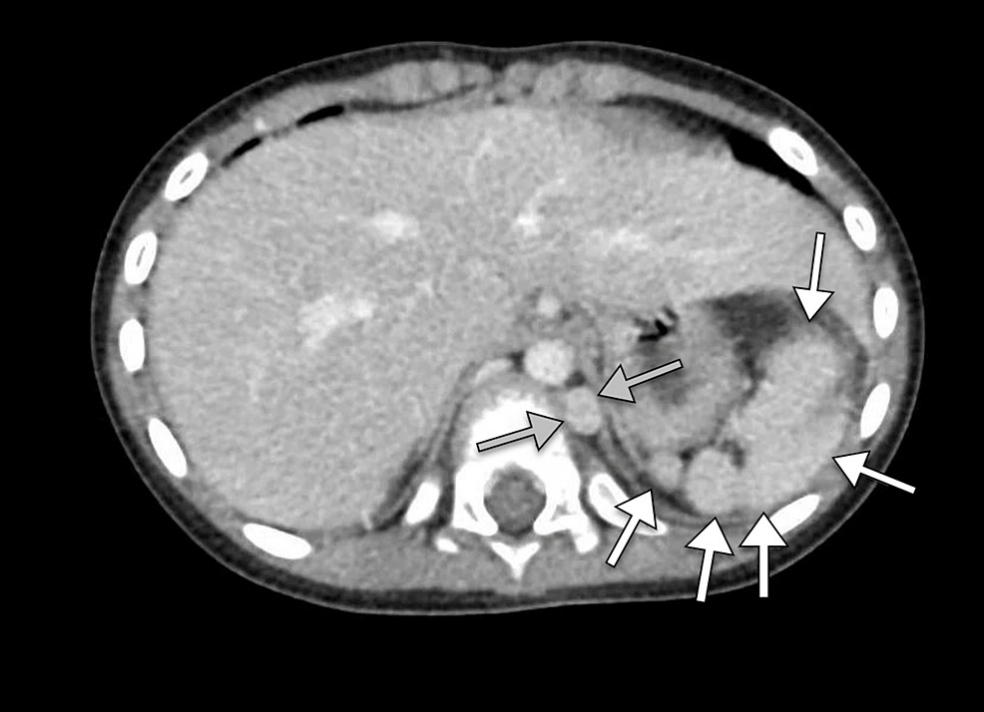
Axial CT image of the abdomen demonstrating multiple spleens (white arrows) and a left-sided inferior vena cava (gray arrows). CT, computed tomography.
